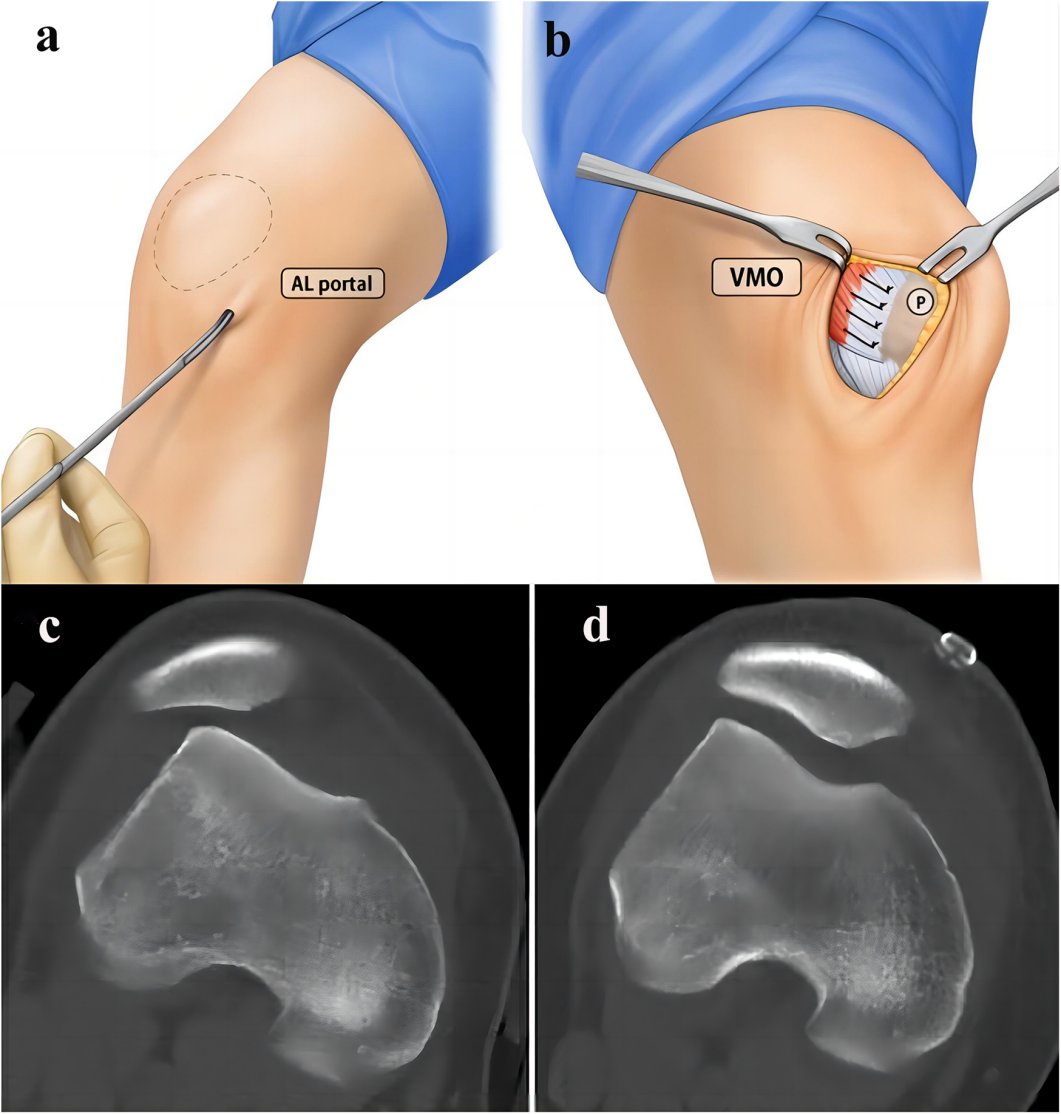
Surgical procedures of the percutaneous LPR release and MPR plication for RPD of the knee. (a) The percutaneous LPR release. (b) The MPR tightening [with permission-Nha et al. (73)]. A 27-year-old female patient who underwent LPR release and MPR plication. (c) Preoperative CT of the knee. (d) Postoperative CT showed that congruence angle, patellar tiltangle and lateral patellofemoral angle have been significantly improved [with permission-Wang et al. (78)].
